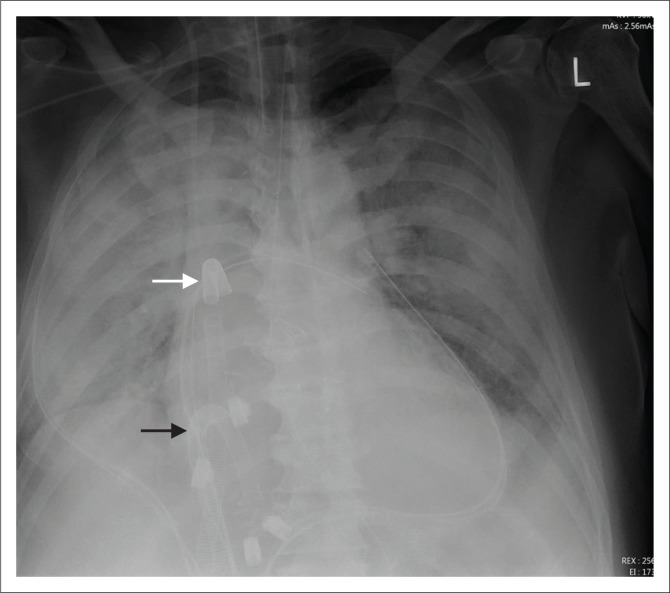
Chest radiograph showing a veno-venous extracorporeal membrane oxygenation with the cannulae in the superior vena cava (white arrow) and right atrium (black arrow) in a 66-year-old male with congestive heart failure (CHF) and pulmonary oedema.
